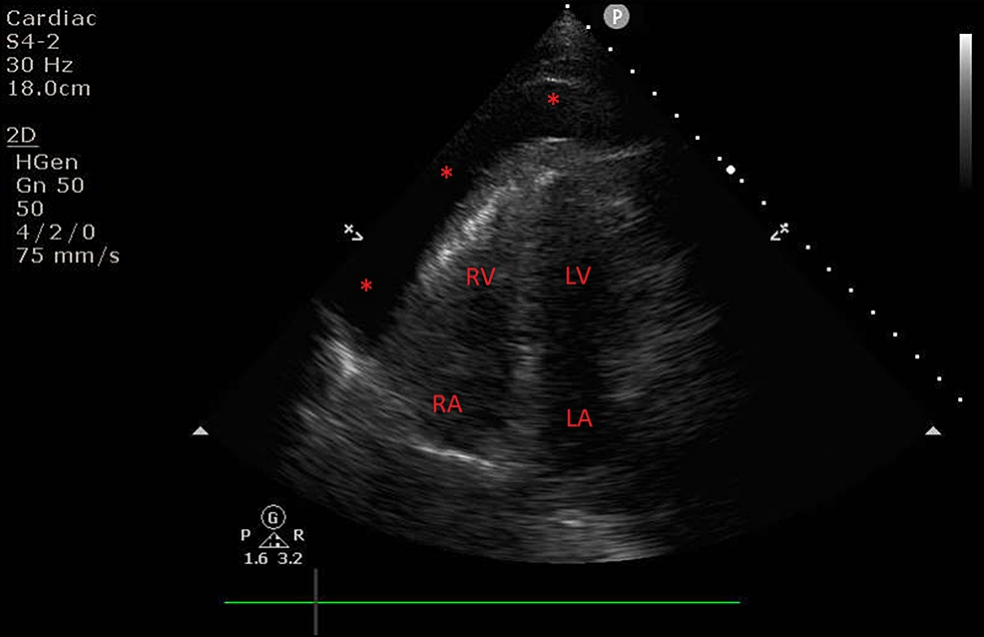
Large pericardial effusion. Transthoracic apical echocardiographic view revealing large pericardial effusion as noted by red asterisks. RA: right atrium; RV: right ventricle; LA: left atrium; LV: left ventricle
